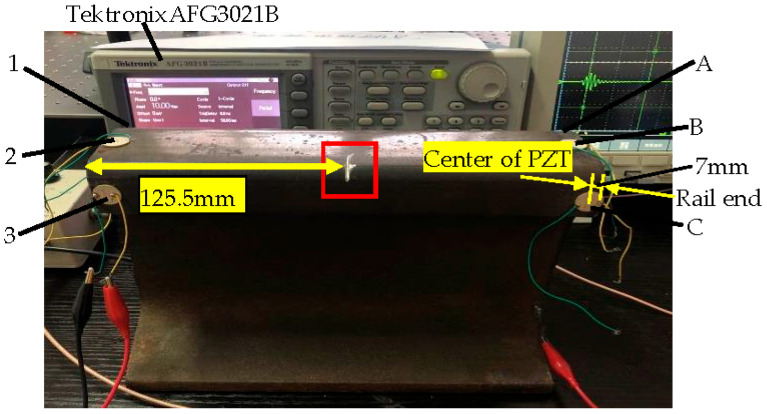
The experimental system for using ultrasonic guided waves detection, in which the position of the red box represents the location of the crack. The PZTs are located at the position of 1, 2, 3, A, B, C, respectively. The distance between the center of PZT and the rail end is 7 mm.
